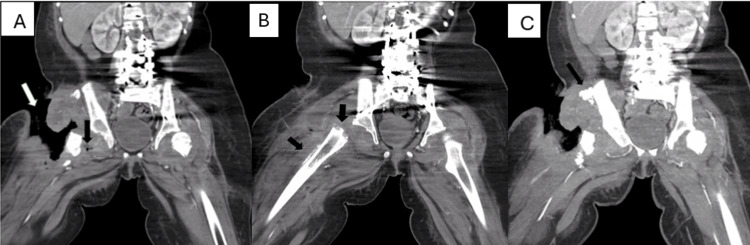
Preoperative coronal computed tomography (CT) images of the bilateral lower extremities, highlighting the extent of the ulcer and anatomical concerns affecting management A) Right inguinal ulcer extending to the proximal femur (white arrow); soft tissue loss and deep soft tissue air medial to the proximal right femur (black arrow). B) Mild periosteal reaction of the right femur (black arrows). C) Heterotopic ossification adjacent to the right iliac crest (black arrow).
